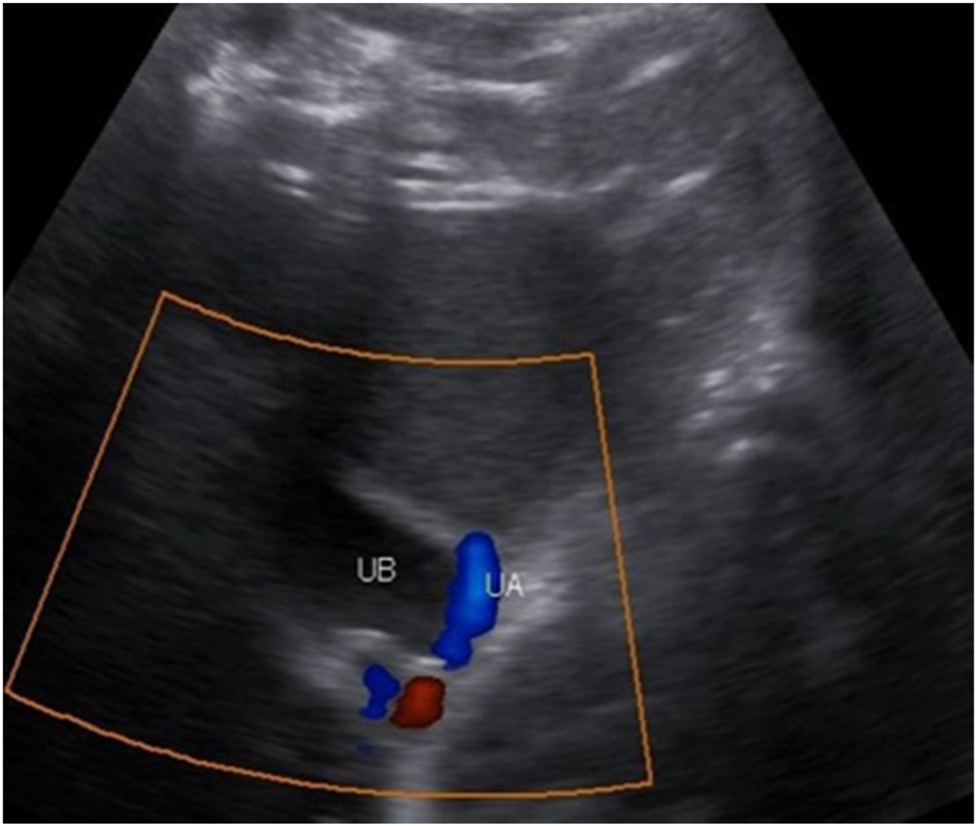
Prenatal obstetric ultrasound showing the fetal urinary bladder with peri vesical umbilical arteries. UB, urinary bladder; UA, umbilical arteries.
